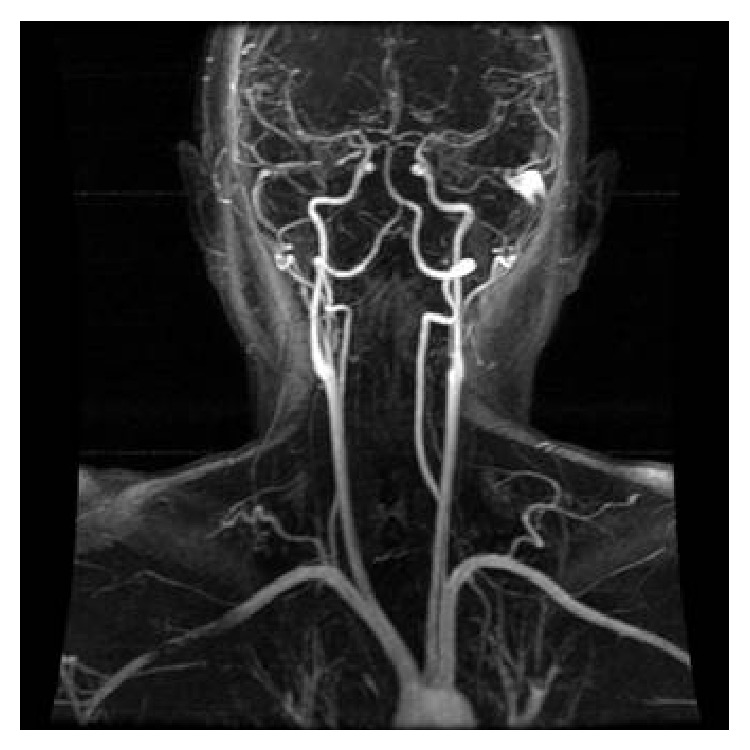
Sagittal view of arterial system (dynamic magnetic resonance image).
